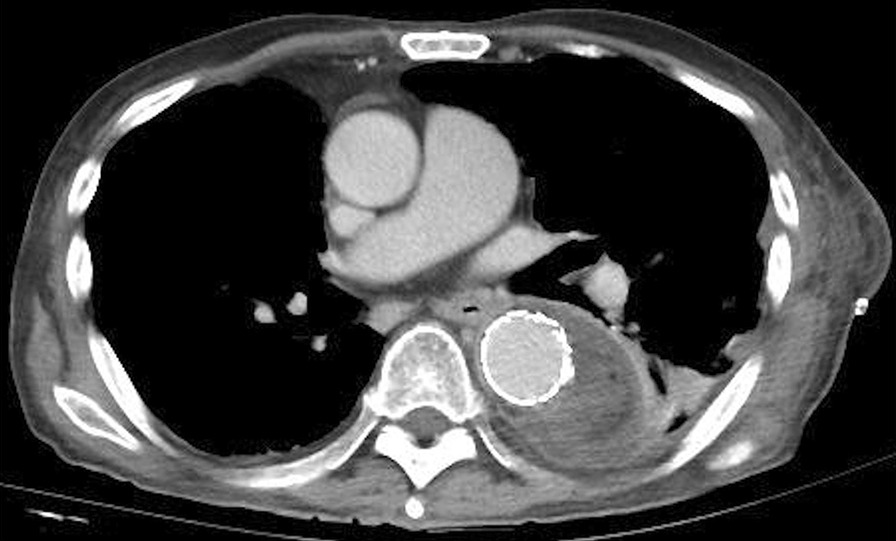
CT performed 7 days after stent graft wrapping confirmed that the LDM flaps were wrapped around the stent graft and that there was no bleeding or other complications
